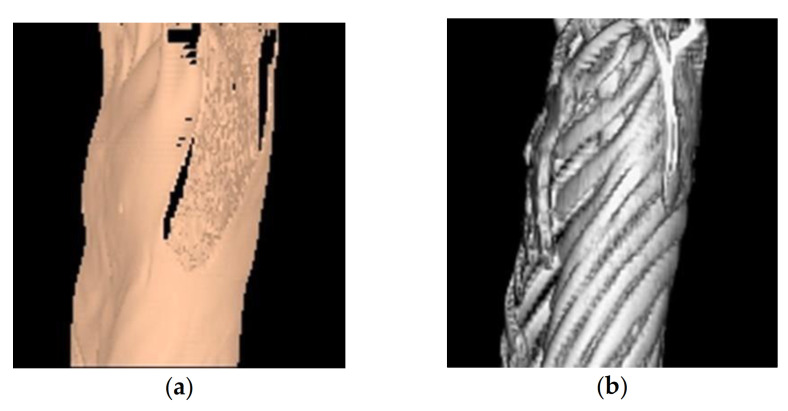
3D-CT of a patient with pleuroparenchymal fibroelastosis. (a) 3D-CT with body surface. Flattening of the chest wall is shown. (b) 3D-CT with bone reconstruction. The intercostal spaces are narrow, and the anteroposterior diameter is shortened. Abbreviation: 3D-CT—three-dimensional computed tomography.
